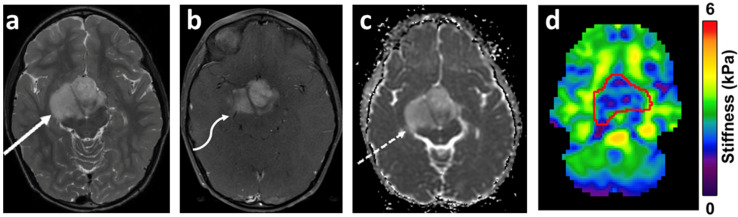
Eight-year-old boy with worsening headaches. Axial T2-weighted image (a) demon-strates a hyperintense, heterogeneous mass centered in the optic-chiasmatic, hypothalamic region (arrow). On the post-contrast T1-weighted image (b) there is moderate enhancement (arrow), with increased diffusivity on the ADC map (c) (arrow). On the stiffness map (d), the mass demonstrates heterogenous decreased stiffness (ROI) as compared to the uninvolved white matter. The tumor stiffness measured 2.48 ± 0.70 kPa, while the average brain stiffness excluding the tumor measured 2.83 ± 0.72. Pathology confirmed a ganglioglioma WHO grade I, with Ki-67: 3–4%. Genetic analysis depicted KIAA1549-BRAF fusion. Author’s institutional human ethics committee/institutional review board guidelines were followed for anonymized images.
